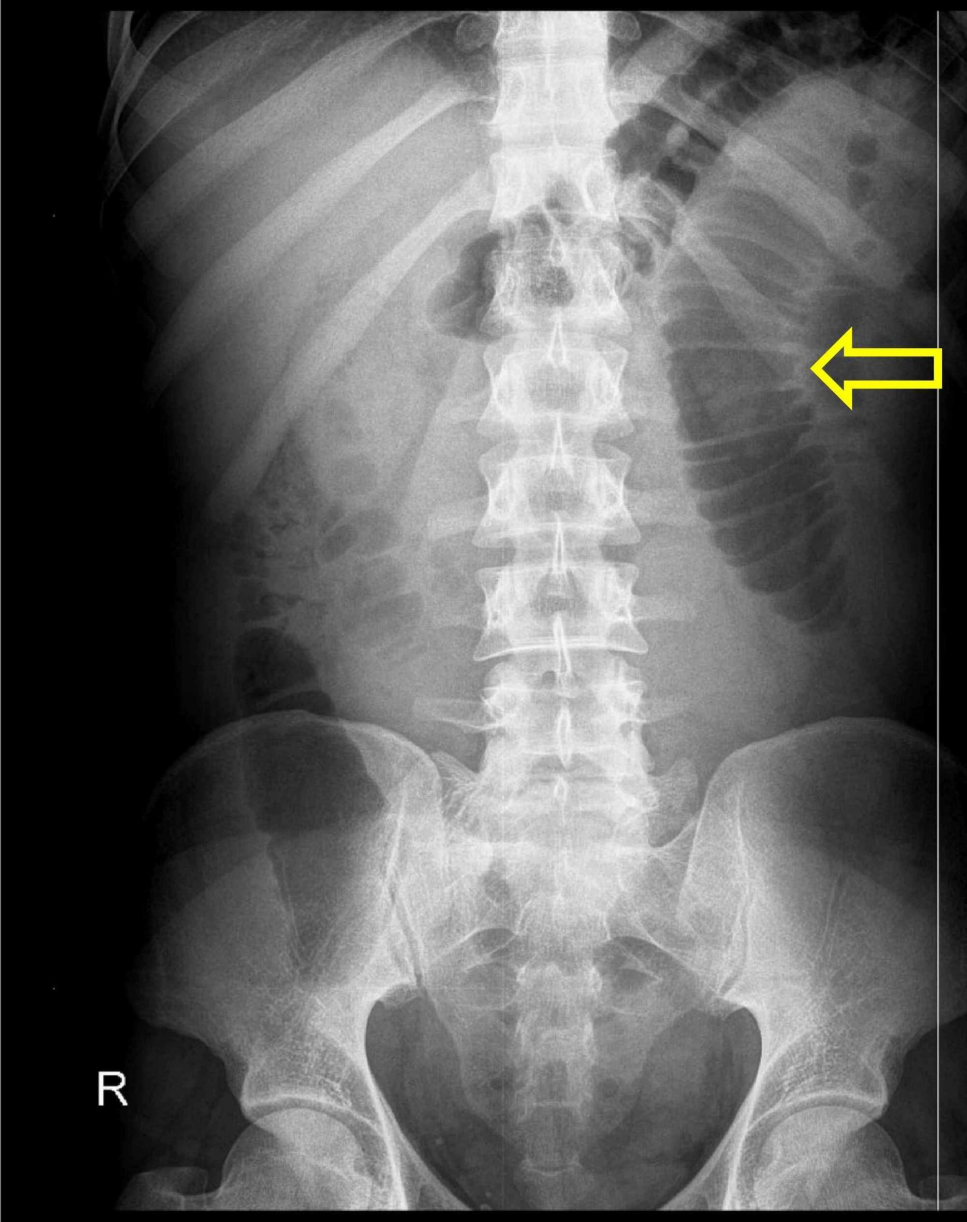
Abdominal X-ray showing dilated jejunum (yellow arrow) with no air in the rectum
